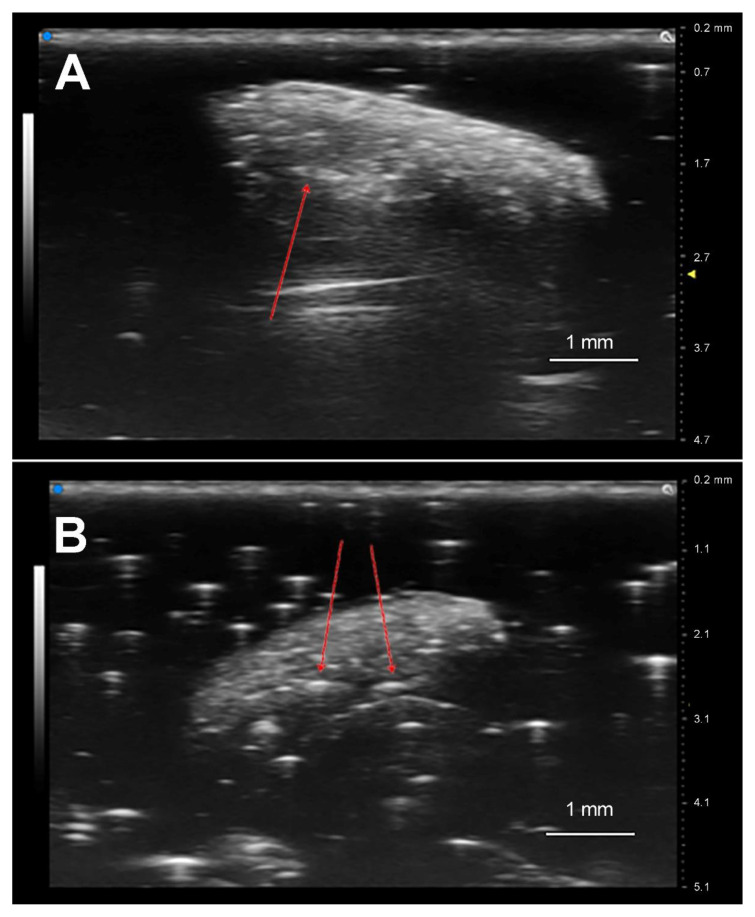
Ultrasound scans, 6 h after parasitization, showing the presence of (A) an egg of the braconid parasitoid *Psyttalia concolor* in a 3rd instar of *Ceratitis capitata* and (B) two eggs of the same parasitoid in a 2nd instar medfly larva.
